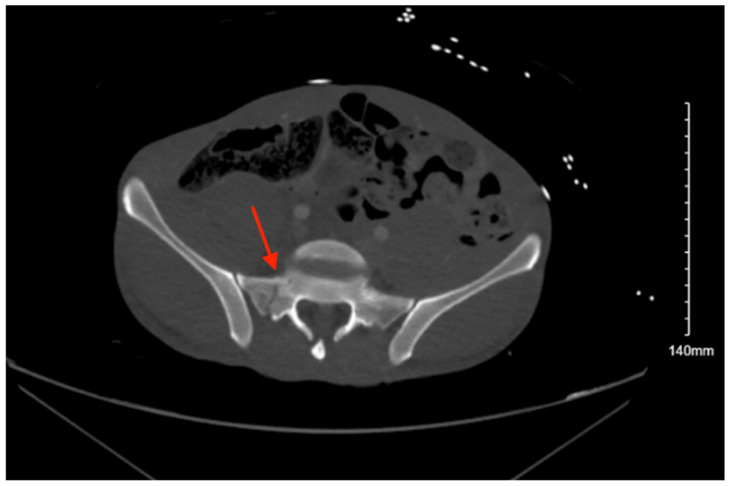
CT abdomen/pelvis images of the described fractures in case 10. Red arrows are used to indicate the location of pelvic fractures for easier identification.
